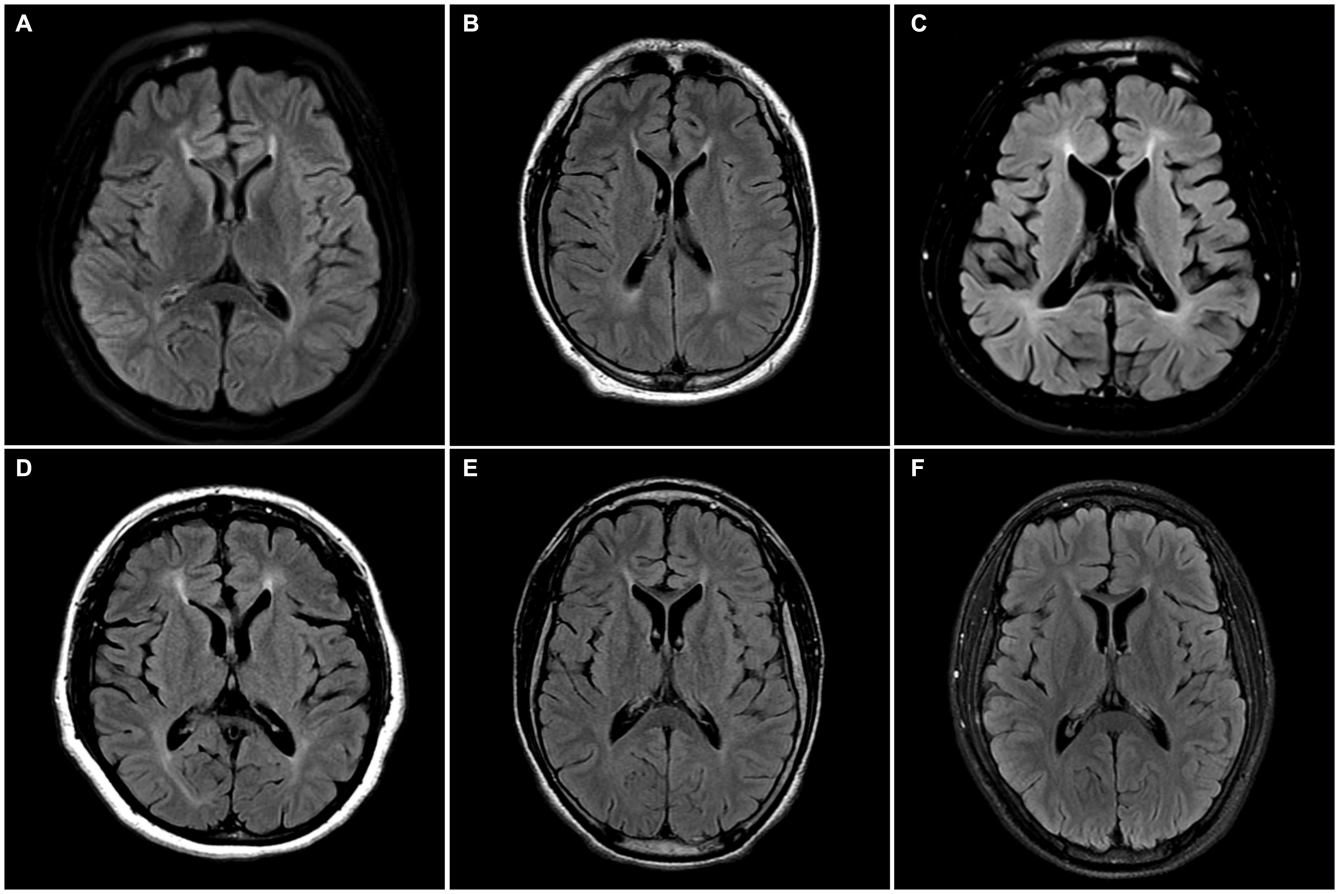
Axial FLAIR MR images of the enrolled patients. (A–F) All subjects have hyperintensities at the forceps minor region called the ears of the lynx sign. (B–D) SN-2, SN-3, and SN-4 have forceps major region signal abnormality. (C) SN-3 has the widening of the lateral ventricle.
